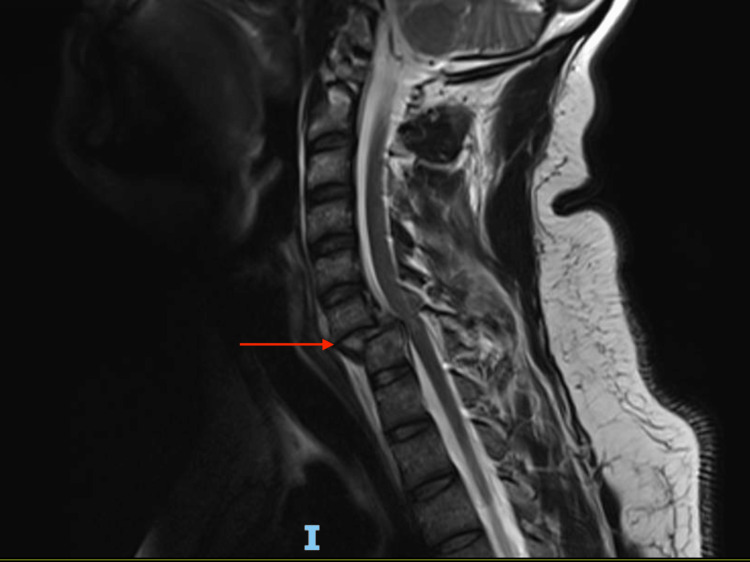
MRI cervical showing C6-C7 fracture dislocation
